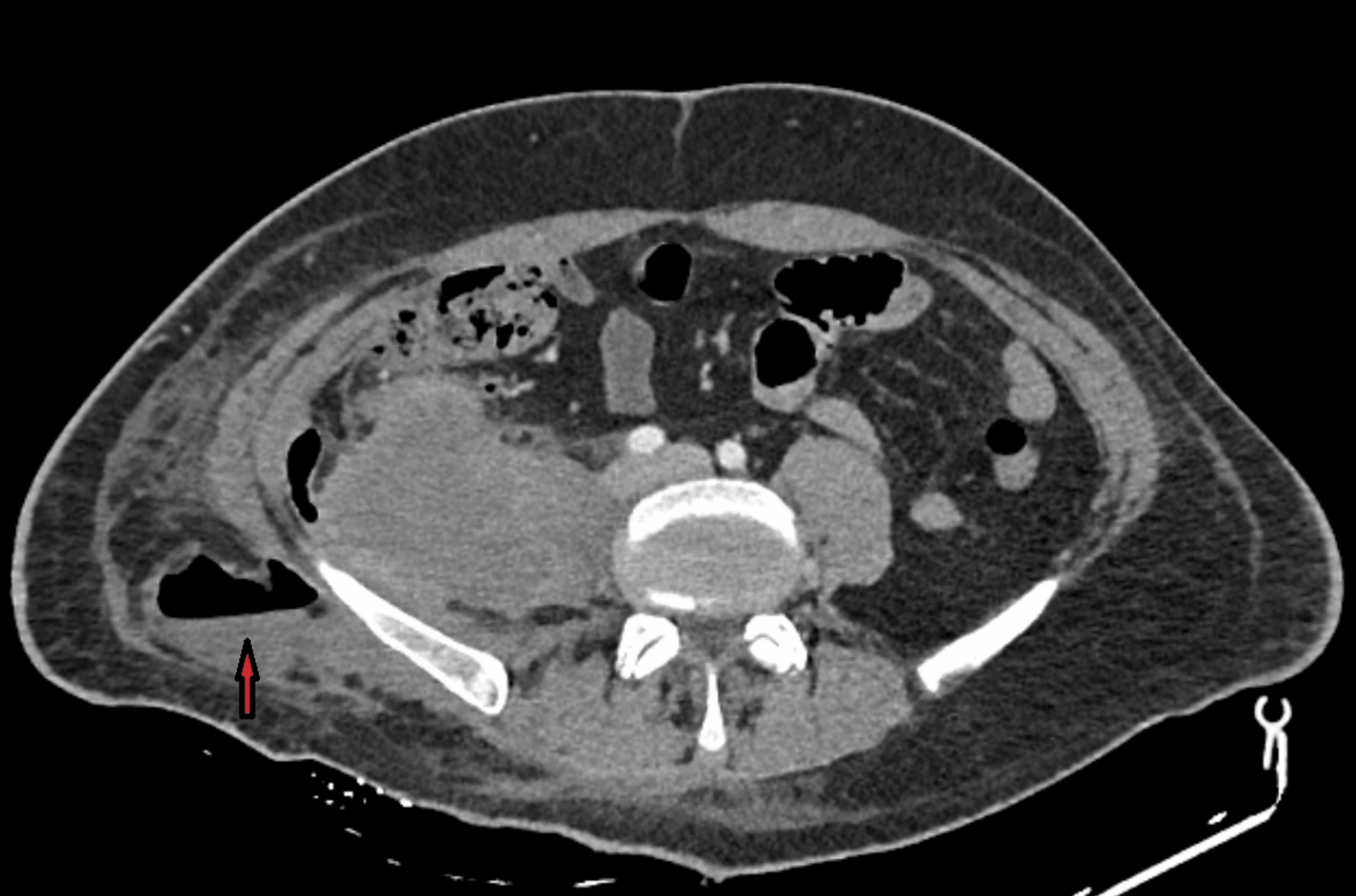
CT findings at presentation revealing the retroperitoneal abscess (red arrow).
